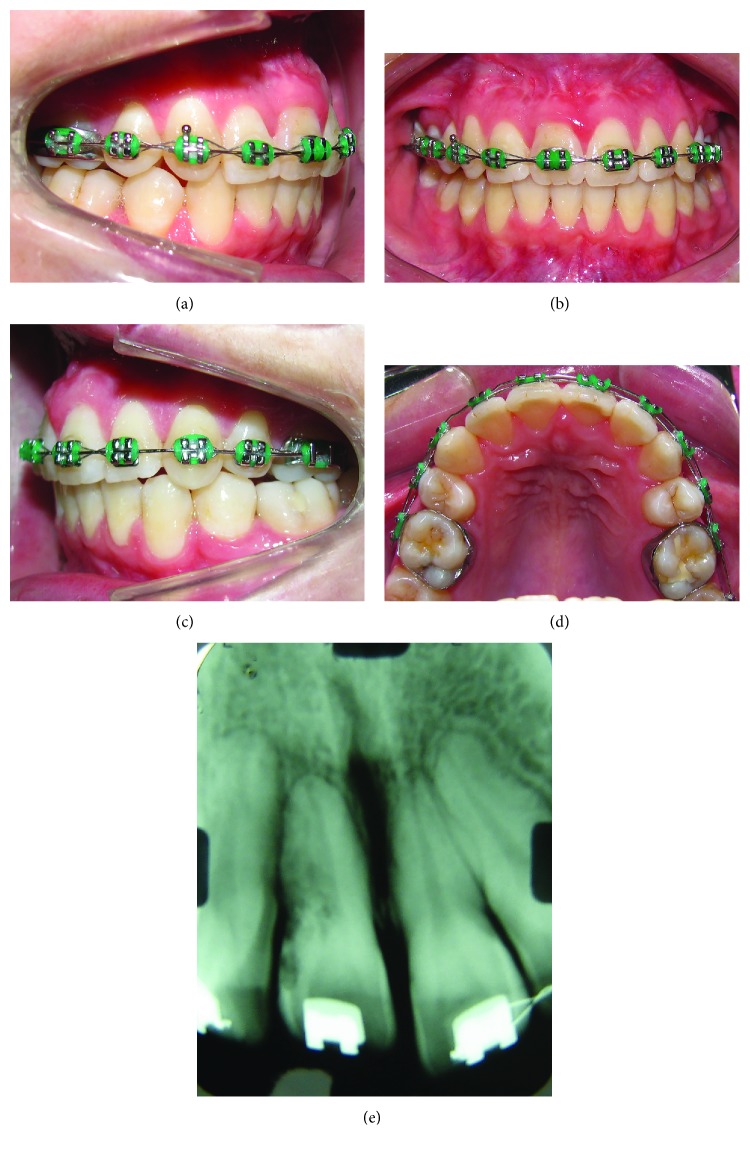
End-of-treatment intrabuccal photographs and periapical radiograph.
